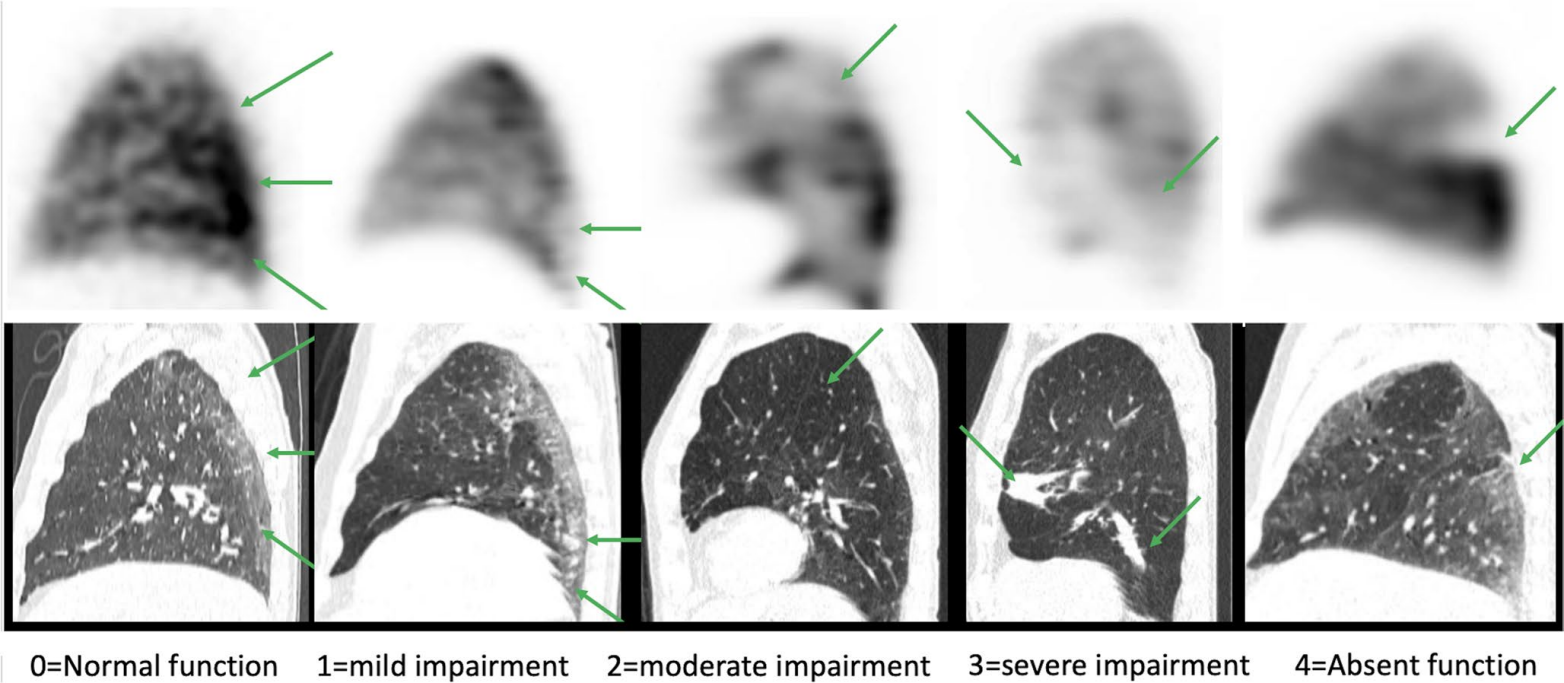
Description of the 5-point scale used for ventilation and perfusion semiquantitative evaluation in a cohort of patient explored for persistent symptom after COVID infection. When CT lesions were visible, perfusion and ventilation were independently evaluated using visual score. Score 0 corresponds to a normal function, 1 to a mild impairment, 2 to a moderate impairment, 3 to a severe impairment, 4 to an absent function. Images are related to pulmonary perfusion scoring related to CT lesions visible (green arrows)
